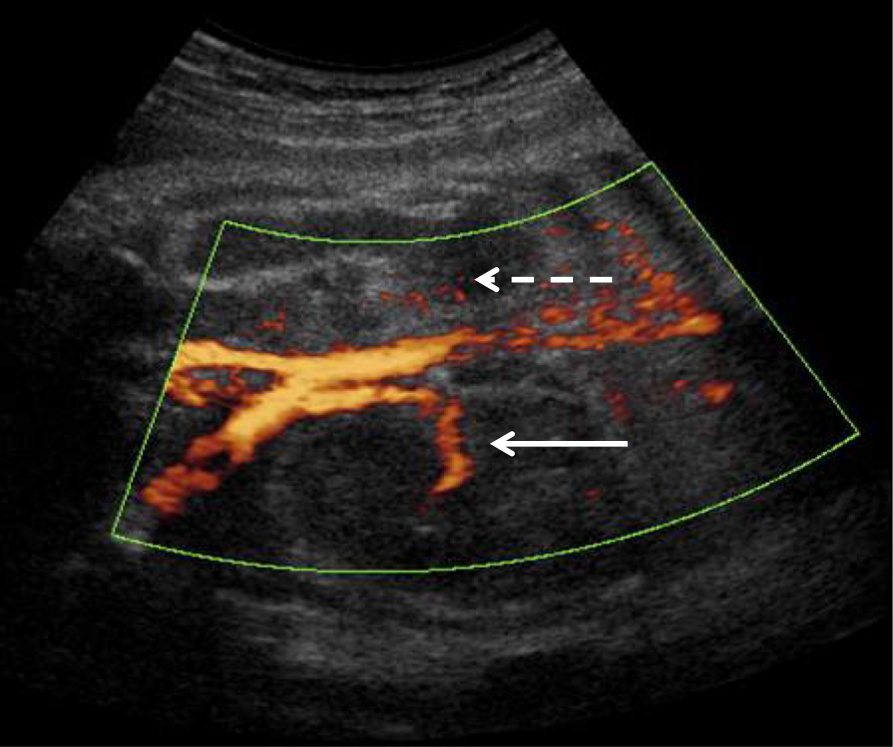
Renal ultrasound at 28 weeks of gestation in the fetus with the p.R257X *KAL1* mutation. The solid white arrow shows the normal right kidney with its pedicle (Doppler US). The dashed white arrow indicates the absence of the left kidney and renal pedicle
